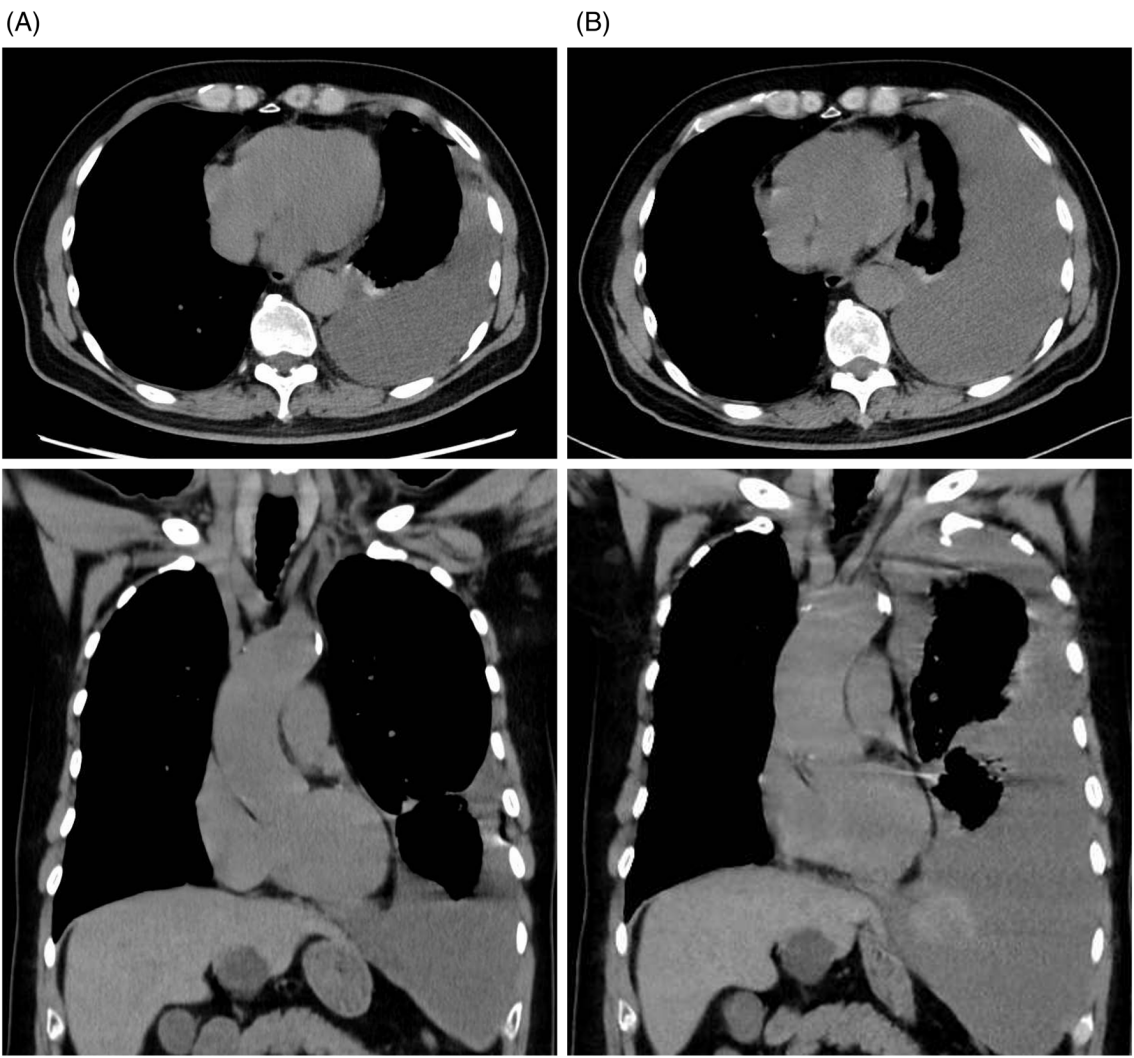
(A) Chest CT image taken by the previous physician at approximately 7–8 h before emergency transport. (B) Chest CT image taken after emergency transport. Increased pleural effusion and mediastinal deviation are observed
